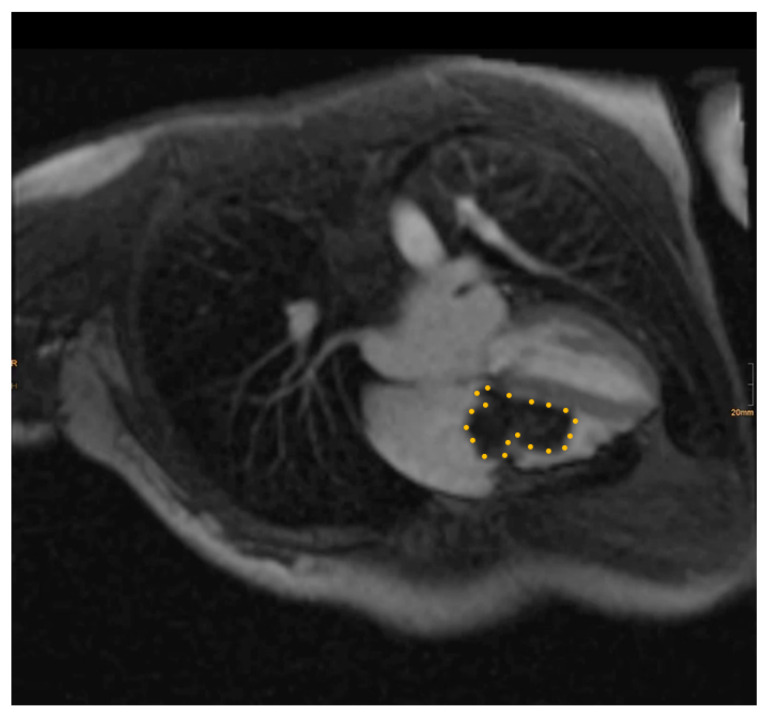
Pre-operative magnetic resonance image. The myxoma boundaries are delineated with a dotted yellow line.
